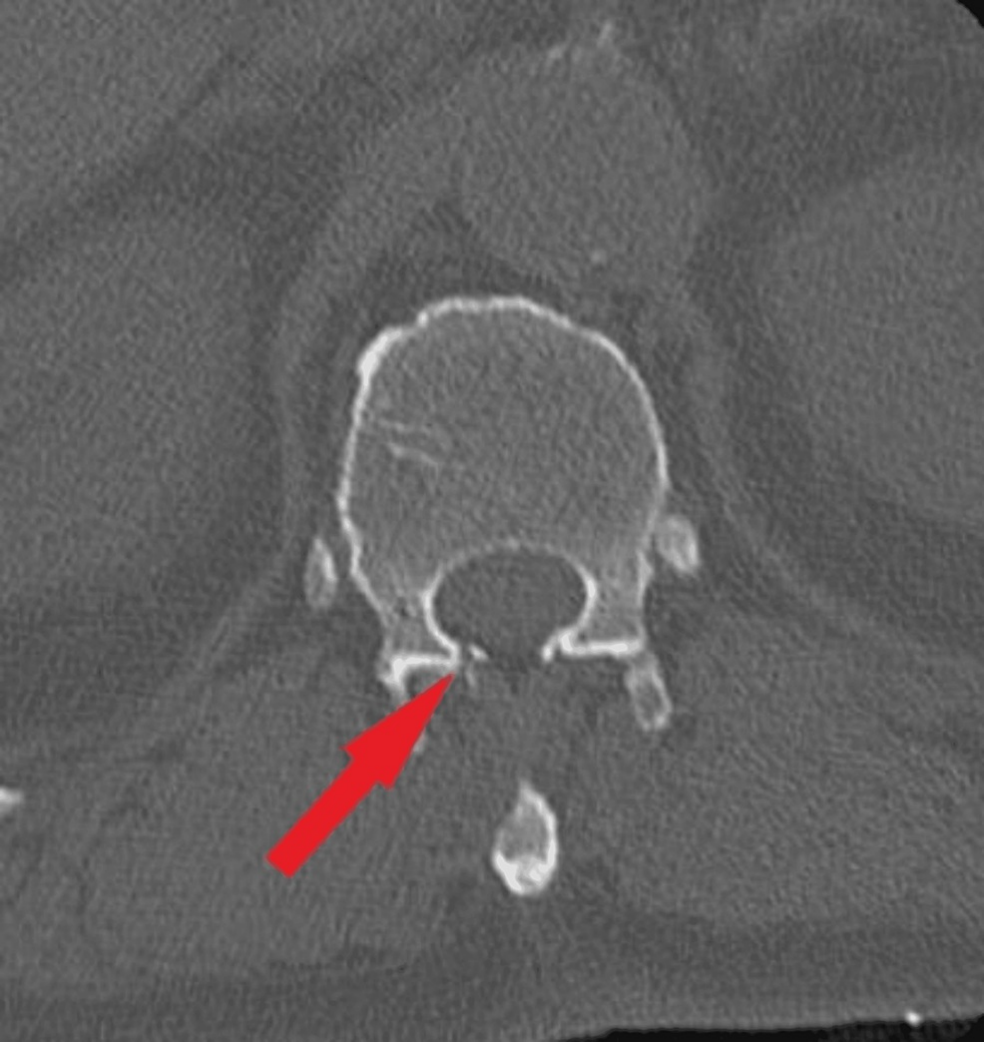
Axial CT scan of lumbar spine, with the red arrow pointing to the epidural catheter with the tip in the epidural space at T11/T12.
